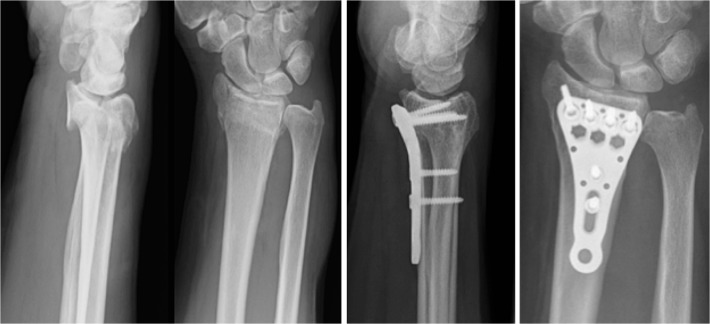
Example of AO 23 C2 fracture lateral and ap (anterior-posterior) view preoperative and 15 weeks postoperative x-rays stabilized with Königsee locking plate.
